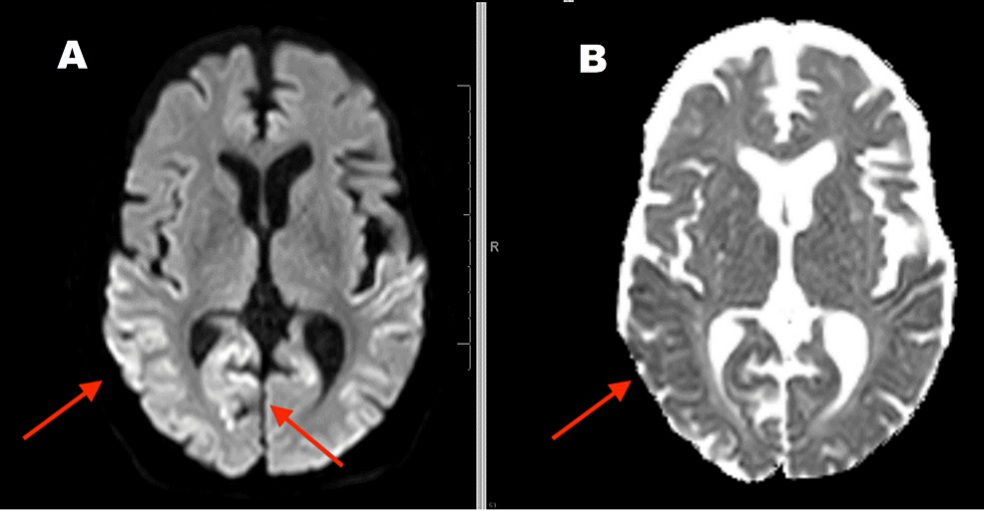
Follow-up MRI Brain after one month shows progression of right parietal and temporal regions with cortical changes in the DWI image (A) (A) Diffusion-weighted imaging (DWI) sequence; (B) Apparent diffusion coefficient (ADC) sequence
